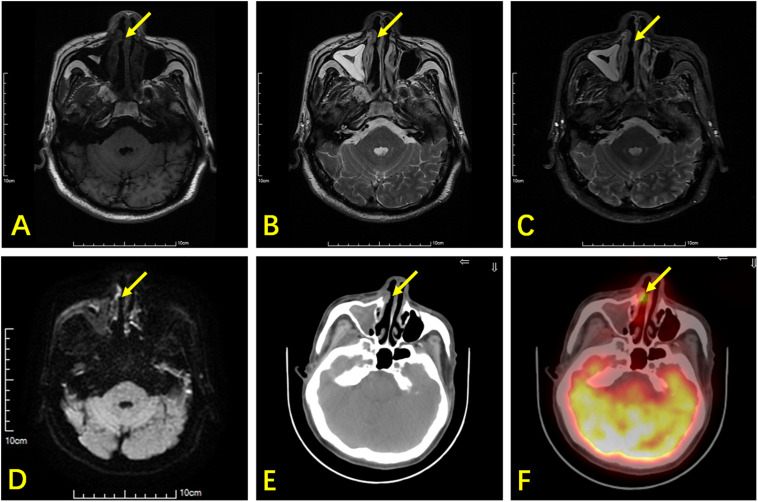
A 66-year-old man with ENKTL. T1WI (A) and T2WI (B) show bilateral thickening in the nasal mucosa with isointensity. (C) Contrast-enhanced T1WI show bilateral heterogeneous enhancement in the nasal mucosa. (D) DWI shows limited lesion diffusion of bilateral nasal mucosa. (E) Axial CT shows a small soft tissue mass (arrow) in the right nasal cavity. (F)
